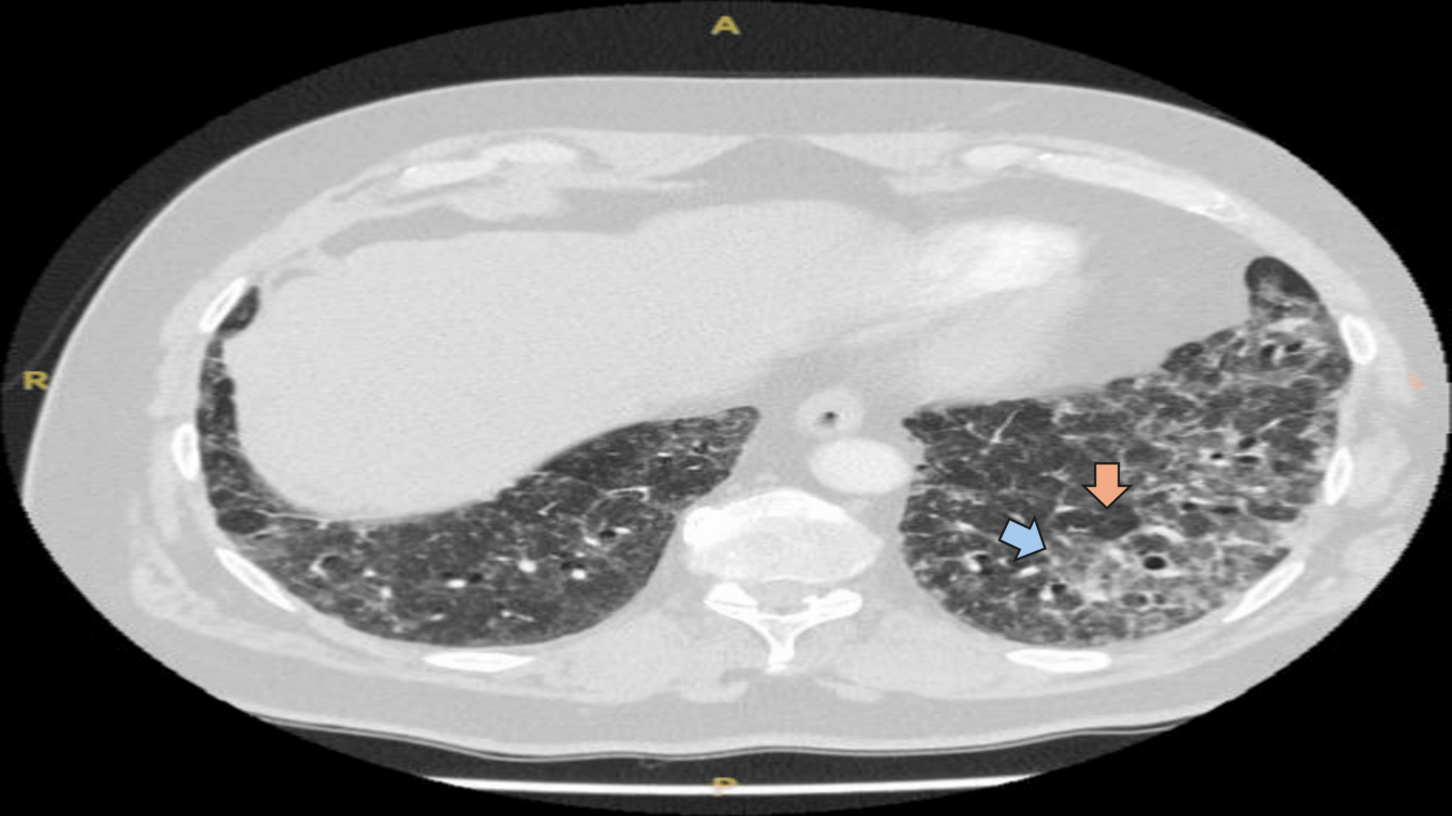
CTPA from current admission Initial CTPA revealing diffuse ground-glass opacities with a centrilobular nodular pattern in both lungs. Additional findings include mosaic attenuation, a tree-in-bud appearance, and areas of air trapping. The blue arrow indicates ground glass opacities. The orange arrow indicates areas of air trapping. CTPA, computed tomography pulmonary angiography
